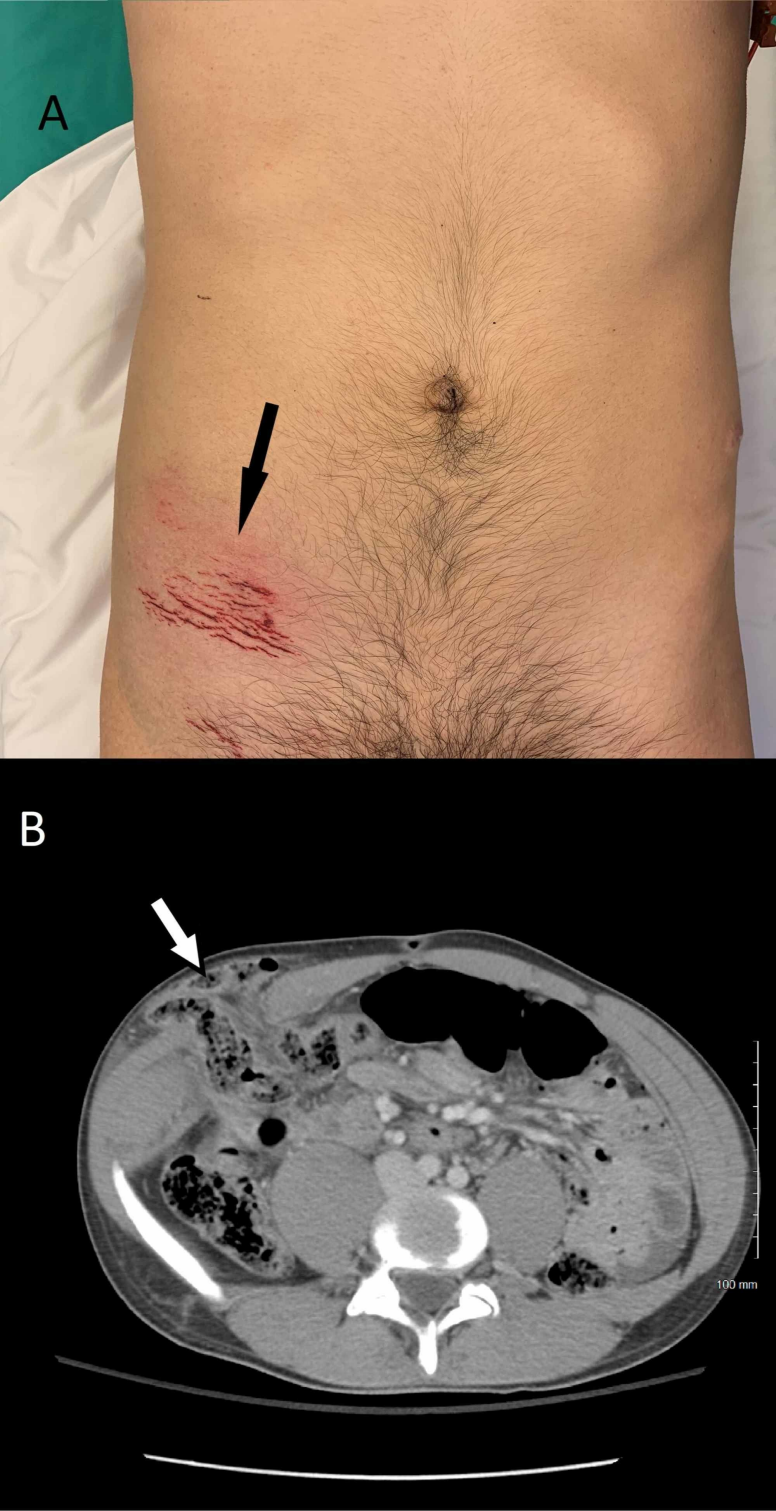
(A) Abrasion and hernia in the right lower quadrant of the abdomen while patient is supine indicated by the black arrow. (B) Contrast-enhanced computed tomography of the abdomen and pelvis which demonstrates a right-sided ventral hernia in the abdominal wall with its content of mesenteric fat and bowel loops indicated by the white arrow.
